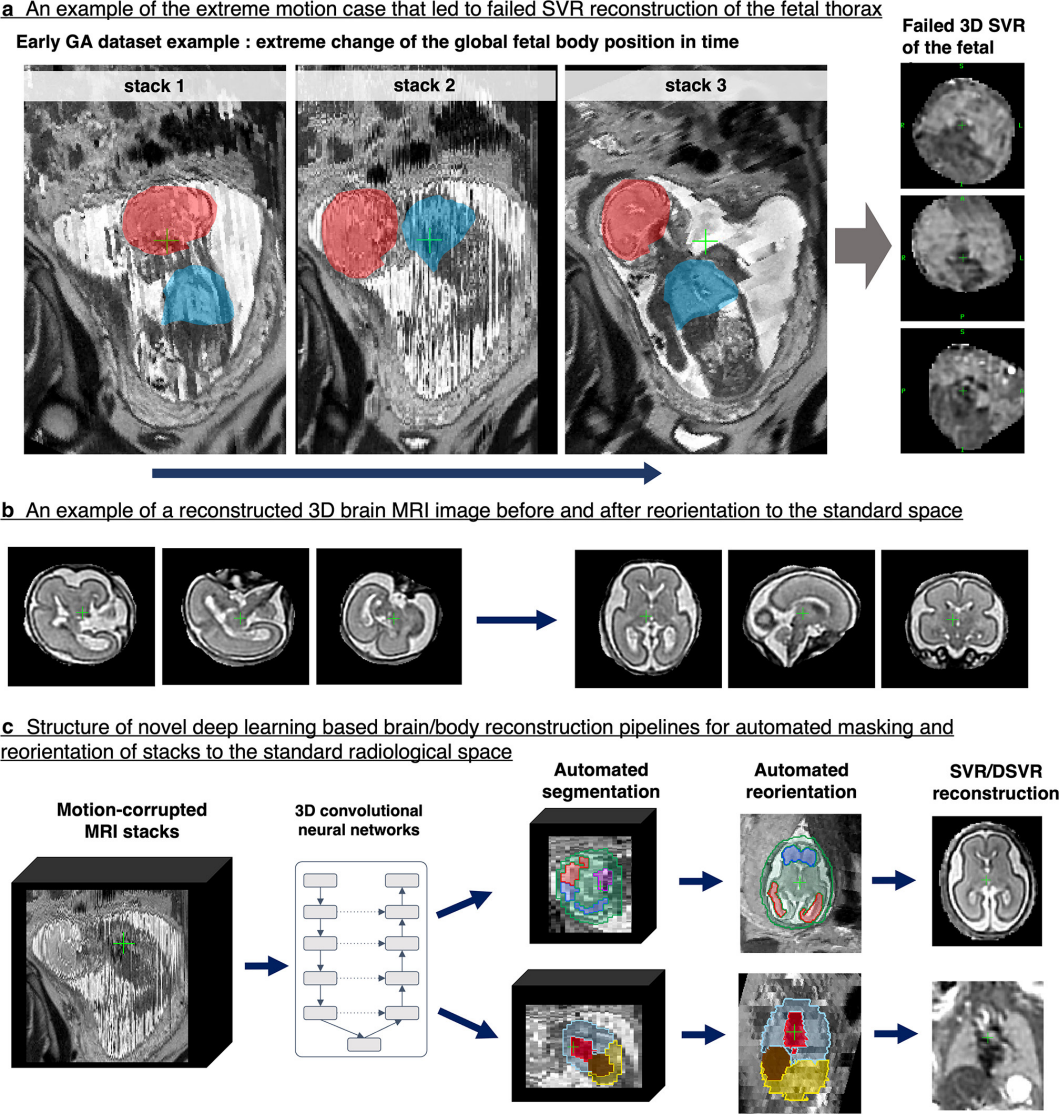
Main limitations of the classical SVR-based methods for foetal MRI and structure of AI-based solutions for automated reconstruction. This example is based on the MRI datasets acquired at St.Thomas’ Hospital, London. AI, artificial intelligence; SVR, slice-to-volume registration.
